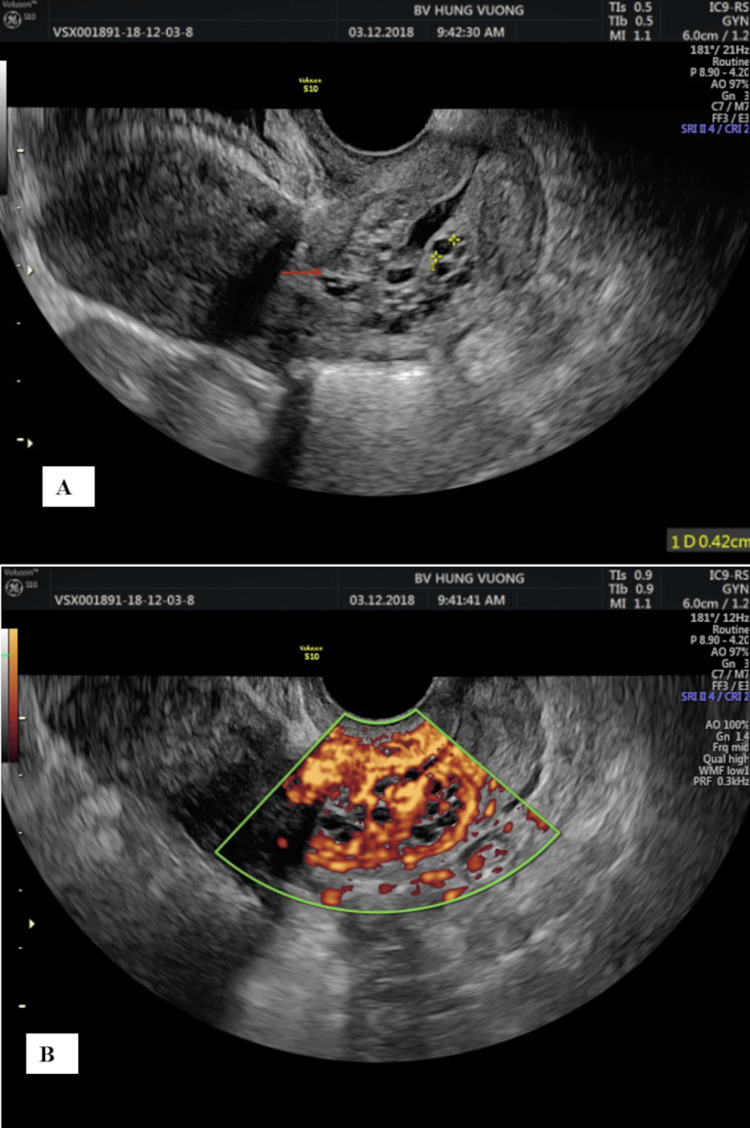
Lesions present in the cervix on 2-D ultrasound images (A) and color ultrasound images (B)
